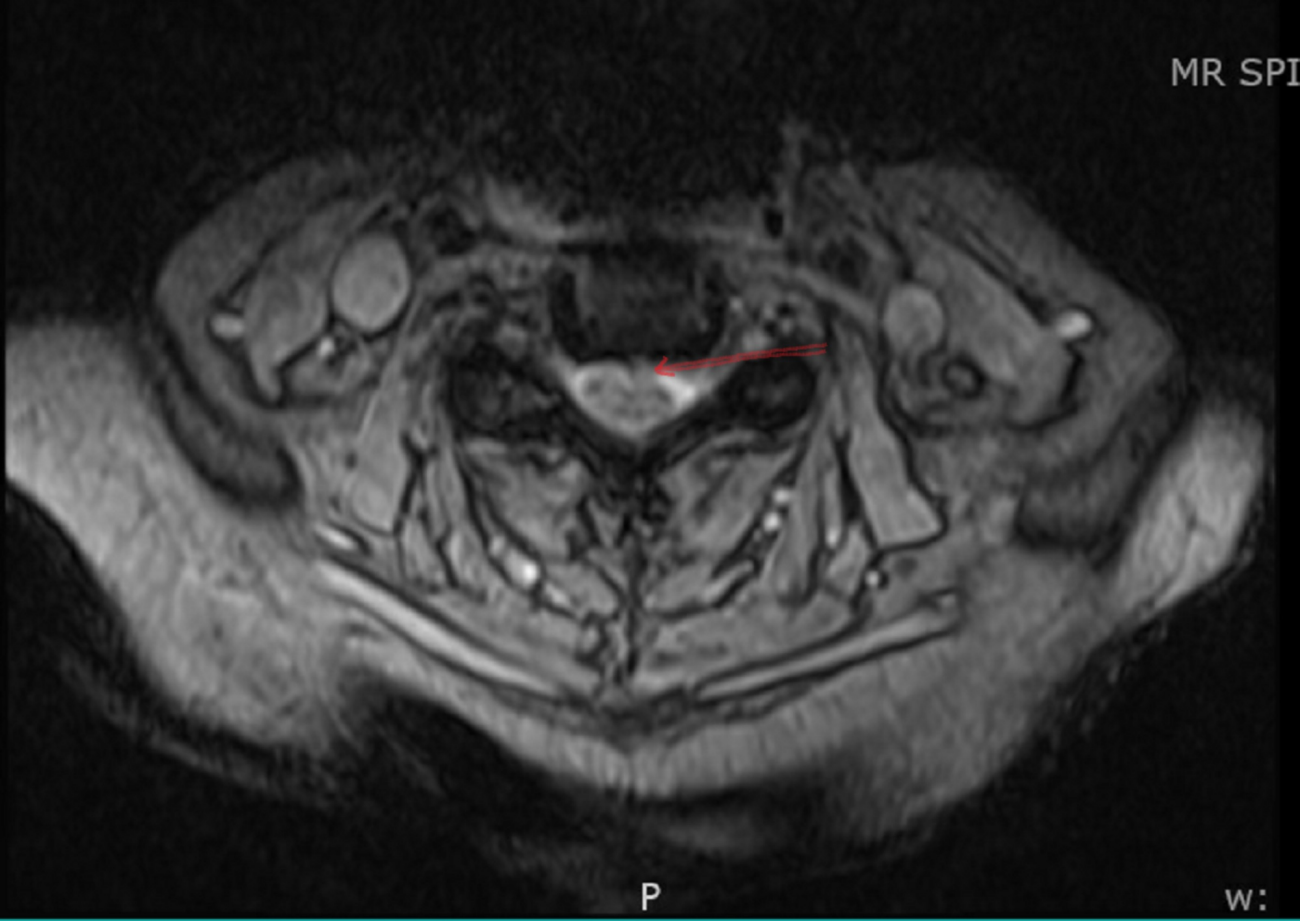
MRI cervical spine showing left side C5-C6 foraminal narrowing (arrow pointing)
